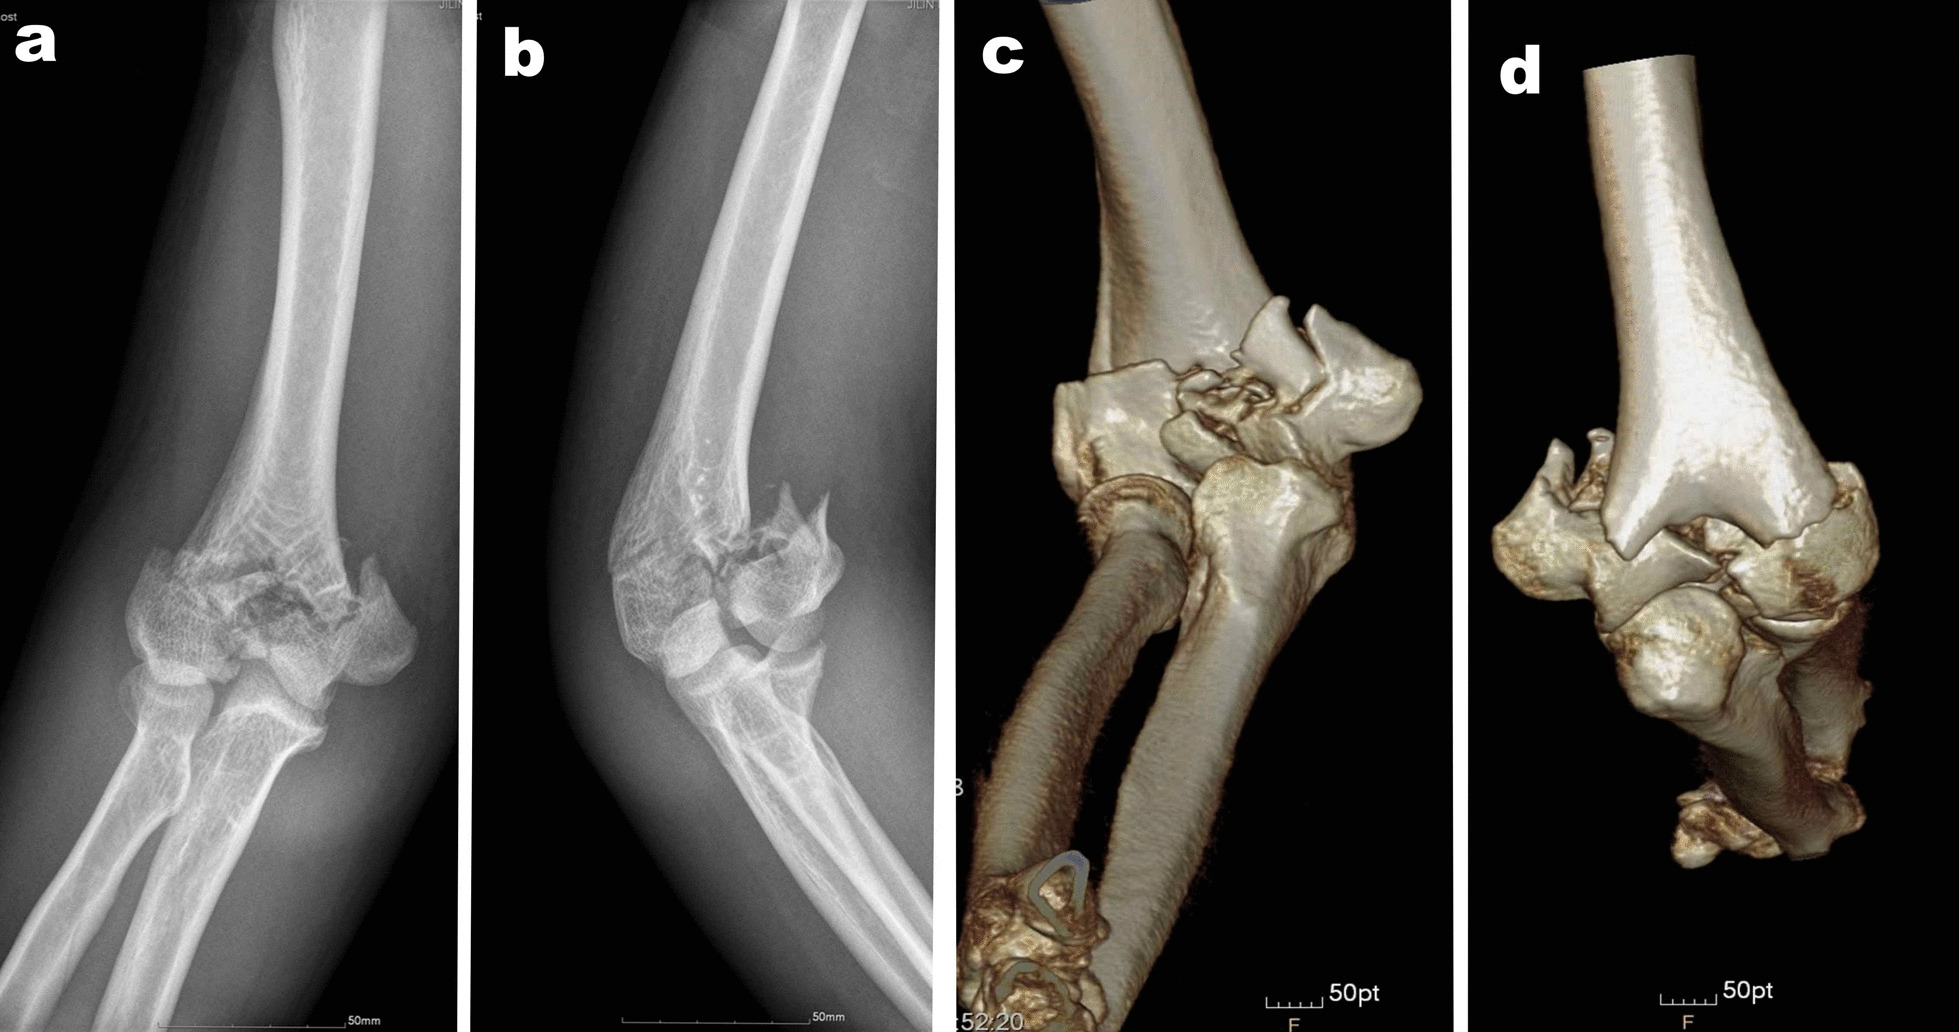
The radiographs of the distal humeral fracture. a–d X-Rays and reconstructed 3D CT images of AO type13C3 distal humeral fracture
